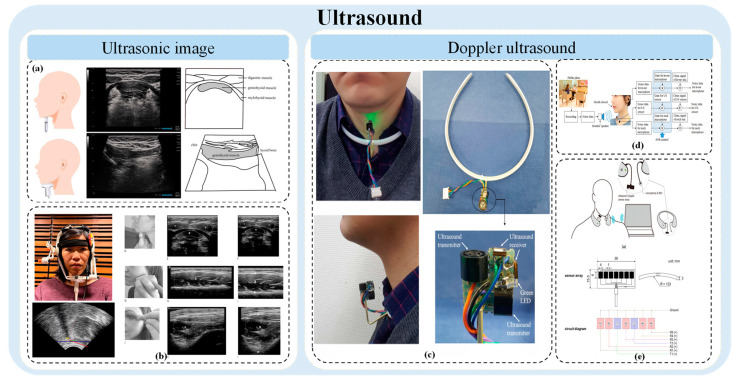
Ultrasound related studies in swallowing assessment. (a) The use of ultrasound in the assessment of eating and swallowing; (b) Ultrasound was used to automatically assess hyoid movement during normal swallowing; (c) Food intake was detected using ultrasonic Doppler sonar; (d) Research on automatic food recognition method combining audio and ultrasonic signals; (e) A swallowing monitoring and evaluation system based on ultrasonic Doppler sensor was developed.
